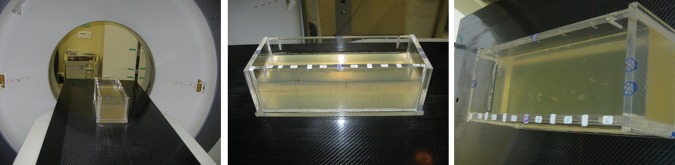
Rectangular box filled with (manufactured) gelatin solution, in which different markers are inserted. Setup for CT scan under standardized conditions (left); box from different views (middle and right).
